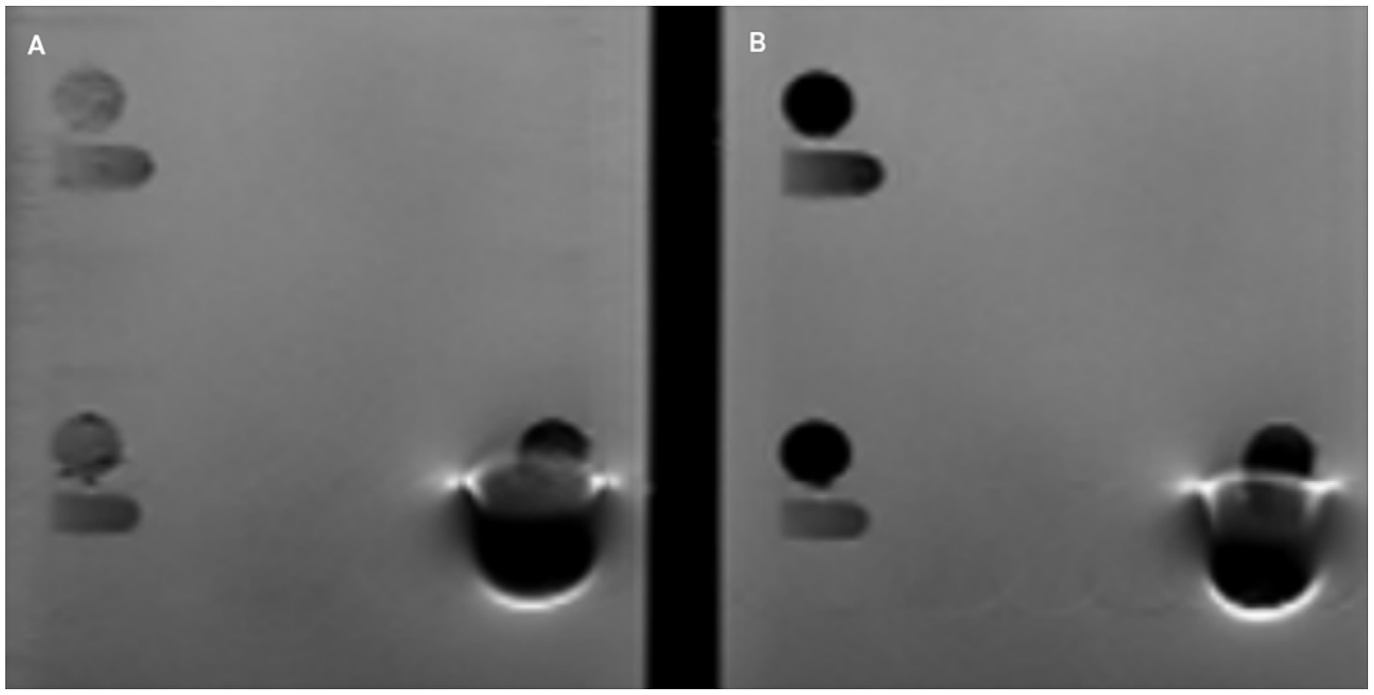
MRI artifact produced by Remanium in (A) T1 and (B) T2.
